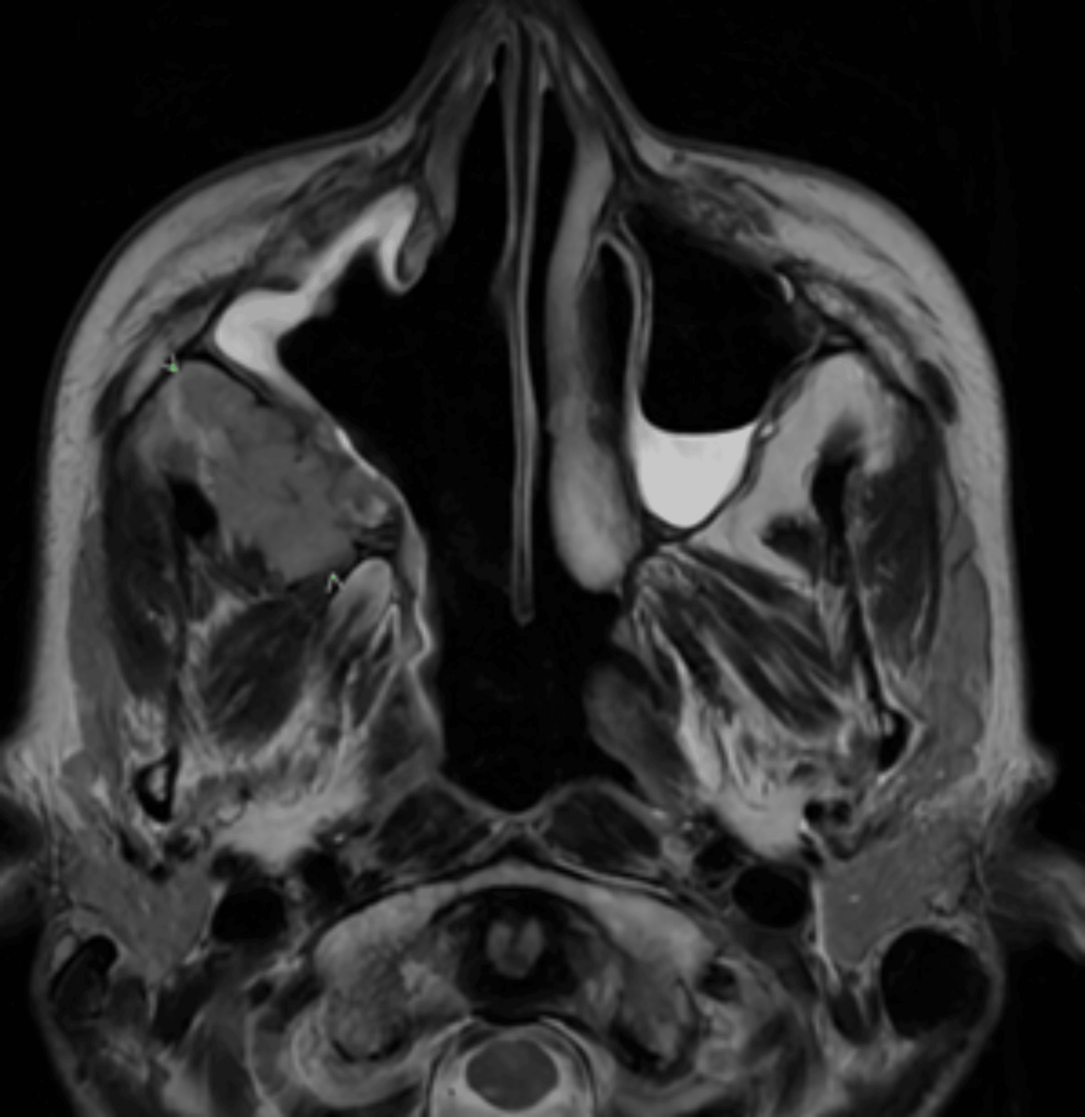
T2-weighted MRI Head and Sinus September 2022 Progressive malignancy with enlarging tumour in the infratemporal fossa (green arrow heads), infiltration into the temporalis, lateral pterygoid and the orbit. Post-surgical changes in the right nasal cavity and medial wall of maxillary sinus can be seen.
